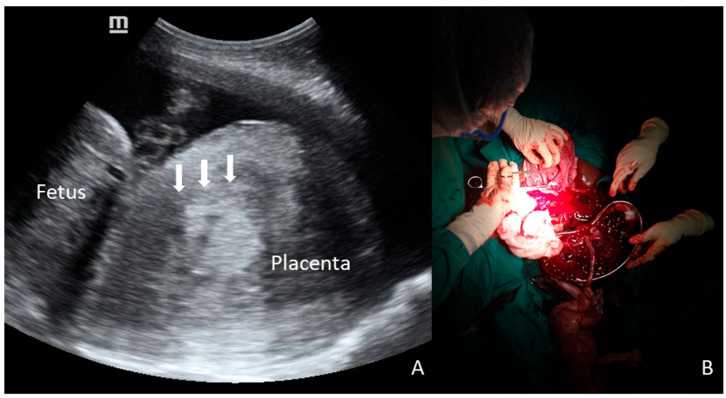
A 28-year-old primigravid woman at 29 weeks of gestation was admitted with antepartum eclampsia. Fetal heart rate was regular upon admission, but POC-US (A) showed an unusual shape of the placenta, represented by a voluminous, round, inhomogeneous, and Doppler-negative mass, raising suspicion of concealed PA (arrows). Despite the proposal for emergency CS, the patient initially refused surgery, and extensive counseling with her family was required. During this period, fetal surveillance was intermittent due to the unavailability of continuous monitoring. After a delay of several hours, CS was performed, and a massive PA was confirmed; the final outcomes were stillbirth and obstetric hemorrhage (800 mL of retroplacental clots, B).
